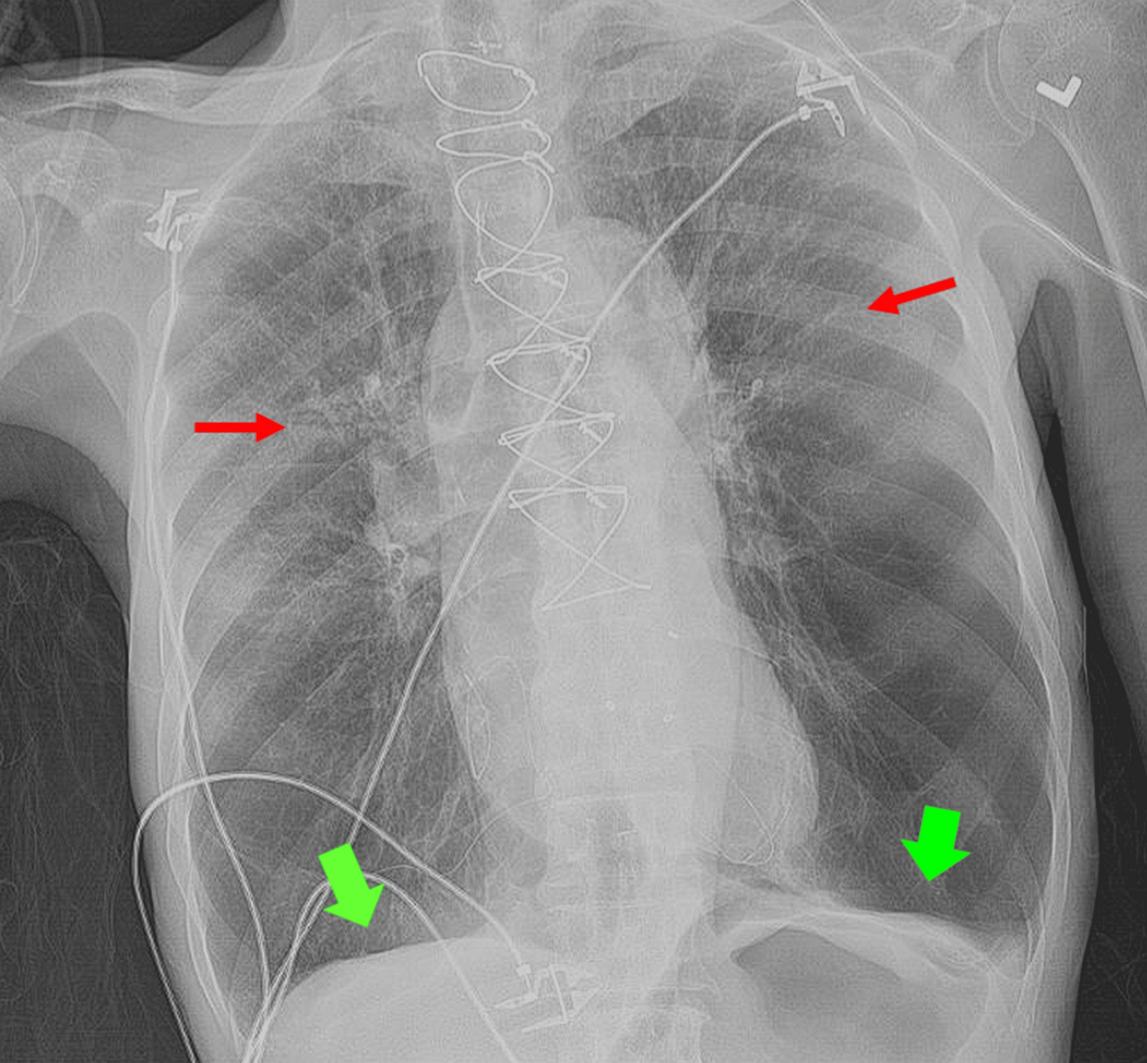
Chest X-ray of the patient on admission. Chest X-ray shows bilateral interstitial opacities (red arrows) and hyperexpansion (green arrows).
